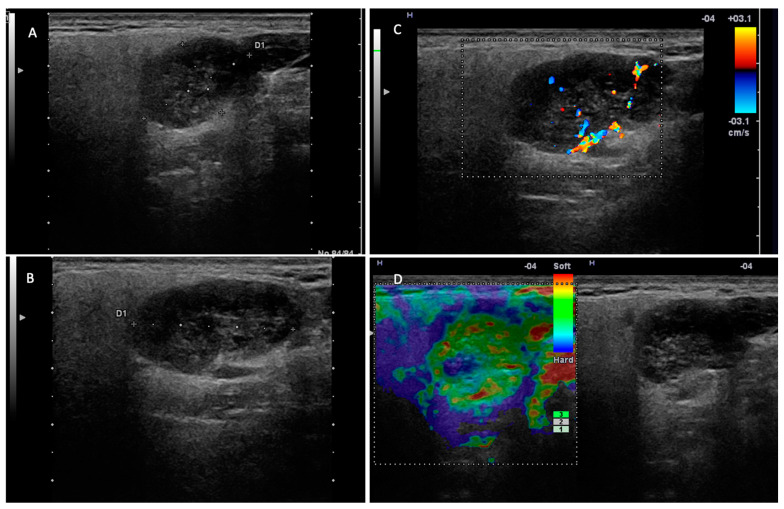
Warthin tumor—right parotid gland. (A,B) Two-dimensional examination revealing a hypoechoic, inhomogeneous nodule with areas of intralesional fluid degeneration, with a slightly irregular contour but well demarcated from the adjacent parotid parenchyma. (C) The color Doppler examination reveals intratumoral vascularization. (D) Elastography examination with peripheral stiffness and intralesional soft appearance.
